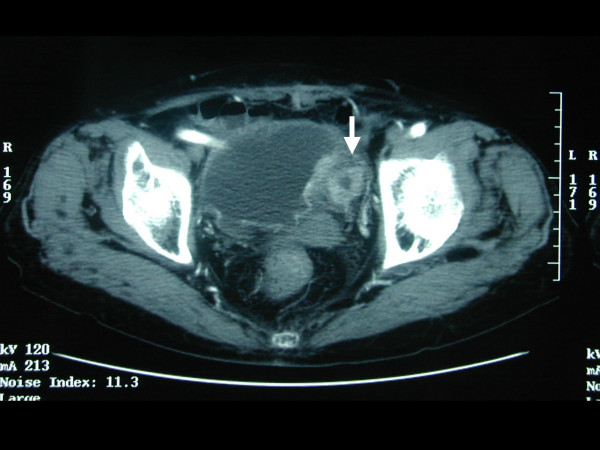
Pelvic computed tomography revealed an abnormally thickened diverticulum of the bladder.
